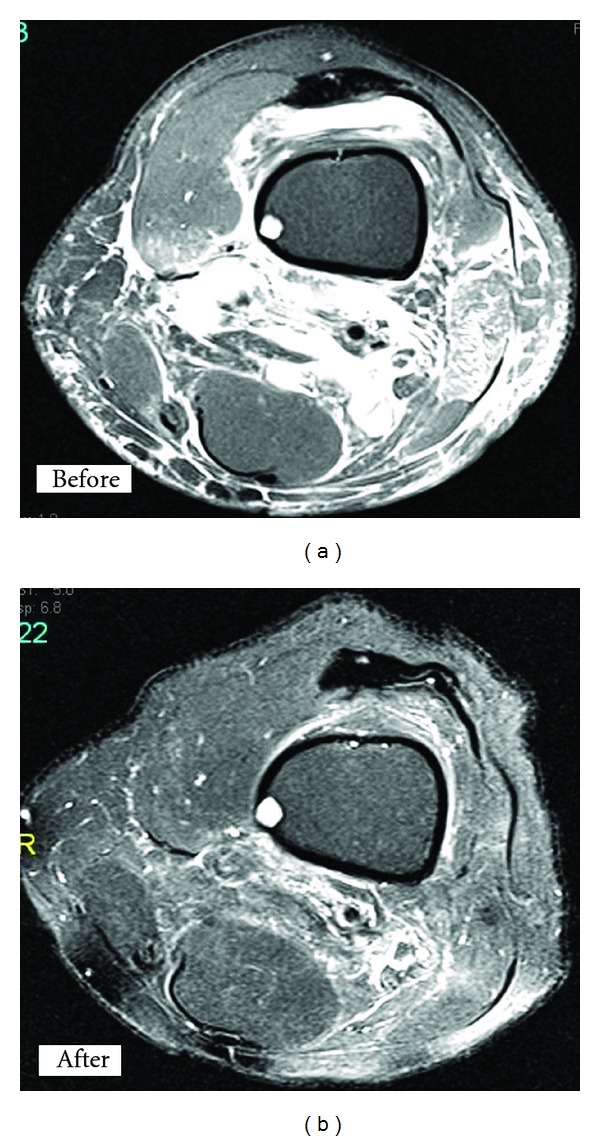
MRI of the Knee showing proximally dissecting ruptured baker cyst before and after treatment.
